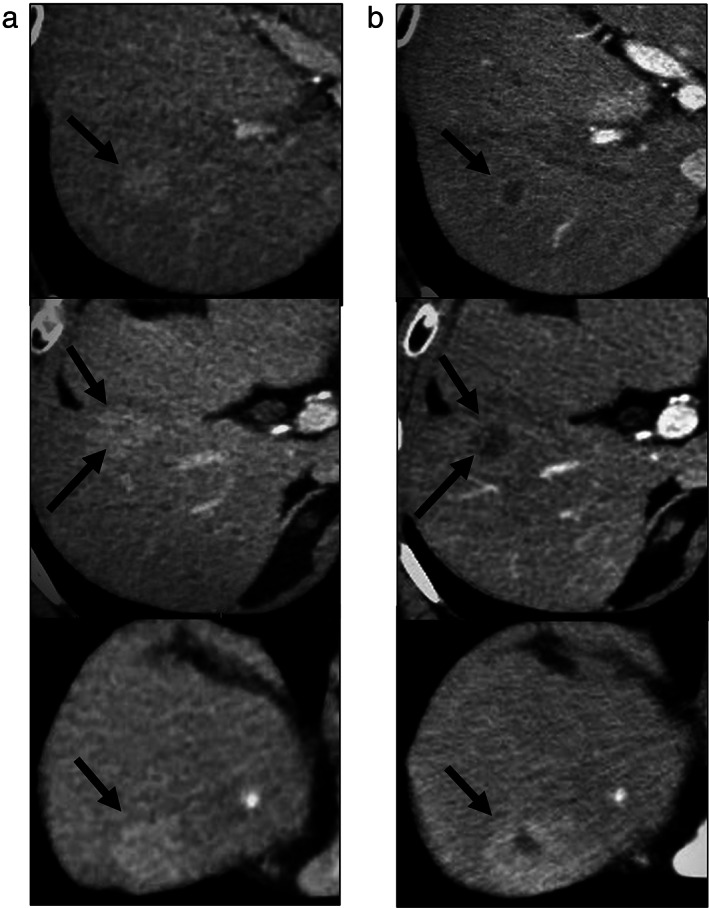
Representative case of a 77‐year‐old male with unresectable hepatocellular carcinoma treated with sorafenib after progression on lenvatinib. (a) A contrast‐enhanced computed tomography examination after lenvatinib failure showed multiple enhanced lesions. (b) Enhancement lesions were decreased at 8 weeks after the administration of sorafenib.
